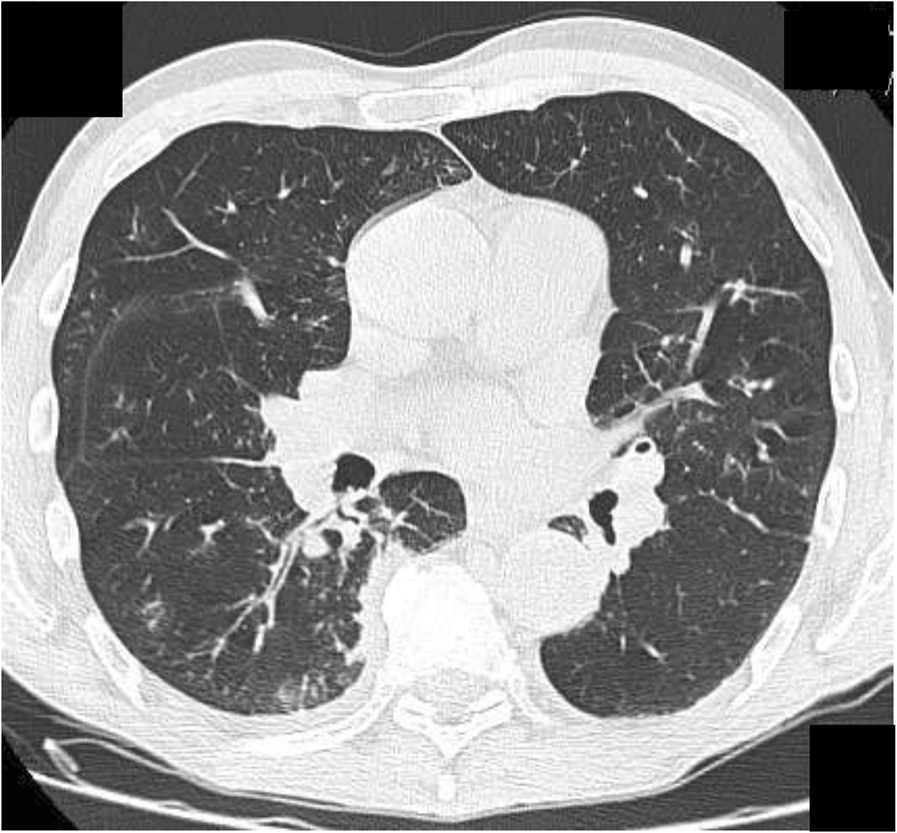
Non-contrast chest computed tomography scan shows multiple bilateral pulmonary infiltrates with ground-glass appearance.
